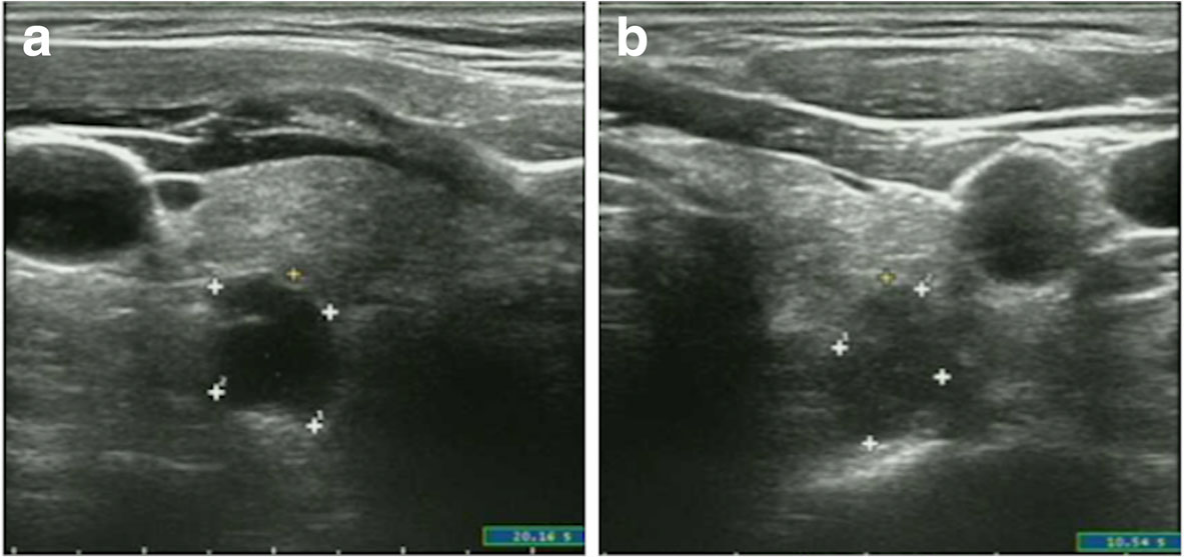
An elderly patient with secondary hyperparathyroidism performed the ultrasonography. The image a showed an enlarged parathyroid gland on the left, with a size of 13.5 mm × 10.8 mm. The image b showed the right enlarged parathyroid gland, with a size of 8.2 mm × 12.8 mm
